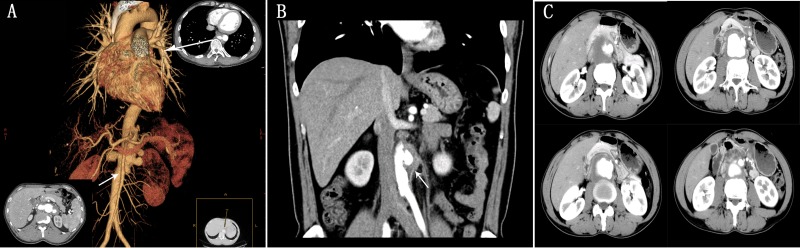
A 35-year-old male with suprarenal abdominal aortic pseudoaneurysm combined with right iliac arterial occlusion (A). Double- bare-metal stent placement combined with coiled embolization to treat the aortic lesion (B), following by balloon angioplasty and self-expansive bare-nitinol stent placement (C). At the 1-week follow-up, three-dimensional volume rendering CT showed obvious attenuation (D), and the lesion was barely detectable at the 2-month, 9-month and 15-month follow-up examinations (E, F, G). As indicated by the red arrow in the images below, a new aneurysm at the proximal end of the bare-metal stent of the iliac artery was detected at the 2-month follow-up examination and its size was stable in the subsequent follow-up examinations under close surveillance.
